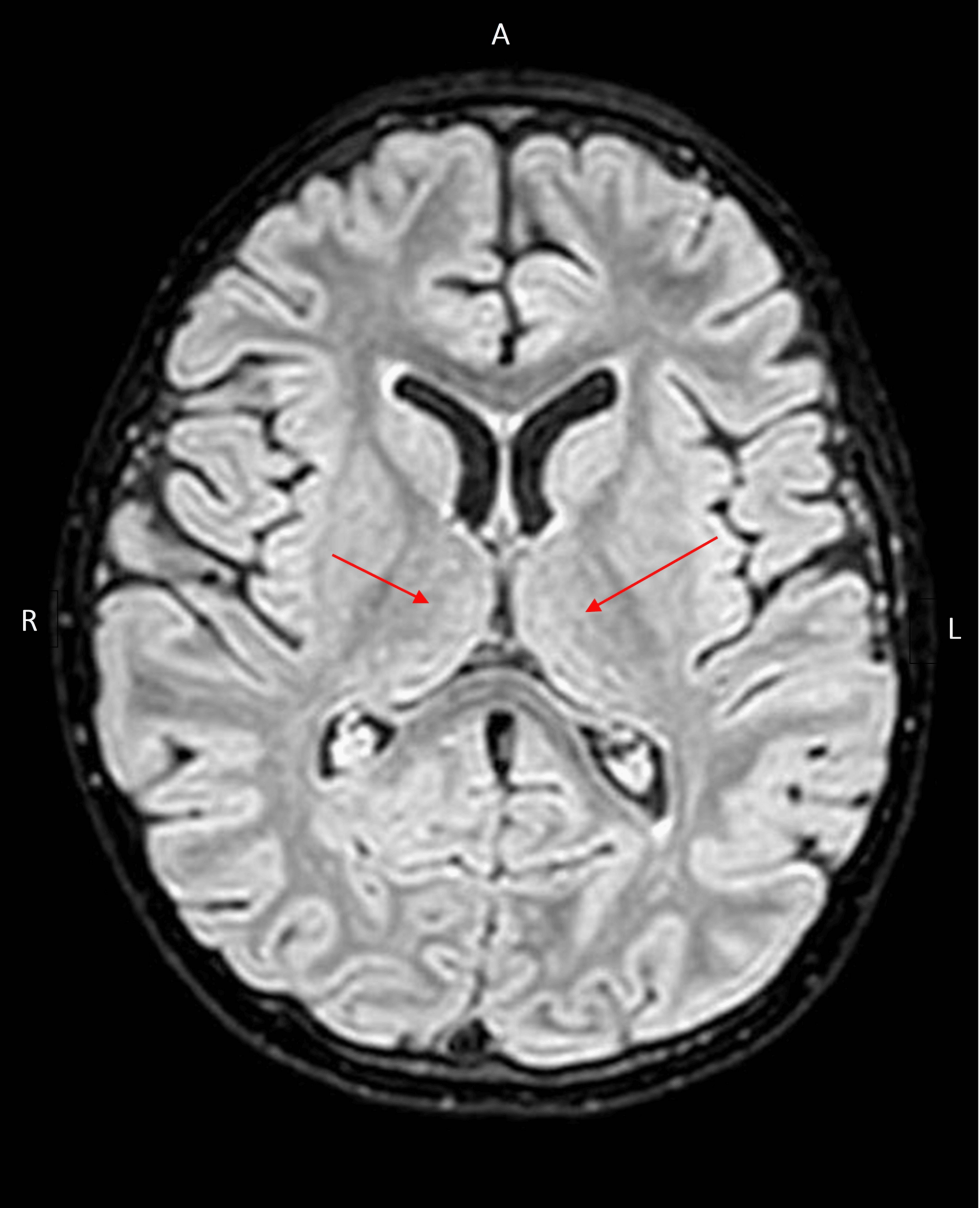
Brain MRI of the patient on admission Axial FLAIR brain MRI showing multiple nonspecific periventricular white matter hyperintense foci (red arrows). No diffusion restriction or abnormal enhancement is seen. The absence of central nervous system lesions helps exclude differential diagnoses such as ADEM, supporting a peripheral etiology consistent with the AMAN subtype of GBS. FLAIR: fluid-attenuated inversion recovery, MRI: magnetic resonance imaging, ADEM: acute disseminated encephalomyelitis, AMAN: acute motor axonal neuropathy, GBS: Guillain-Barré syndrome
